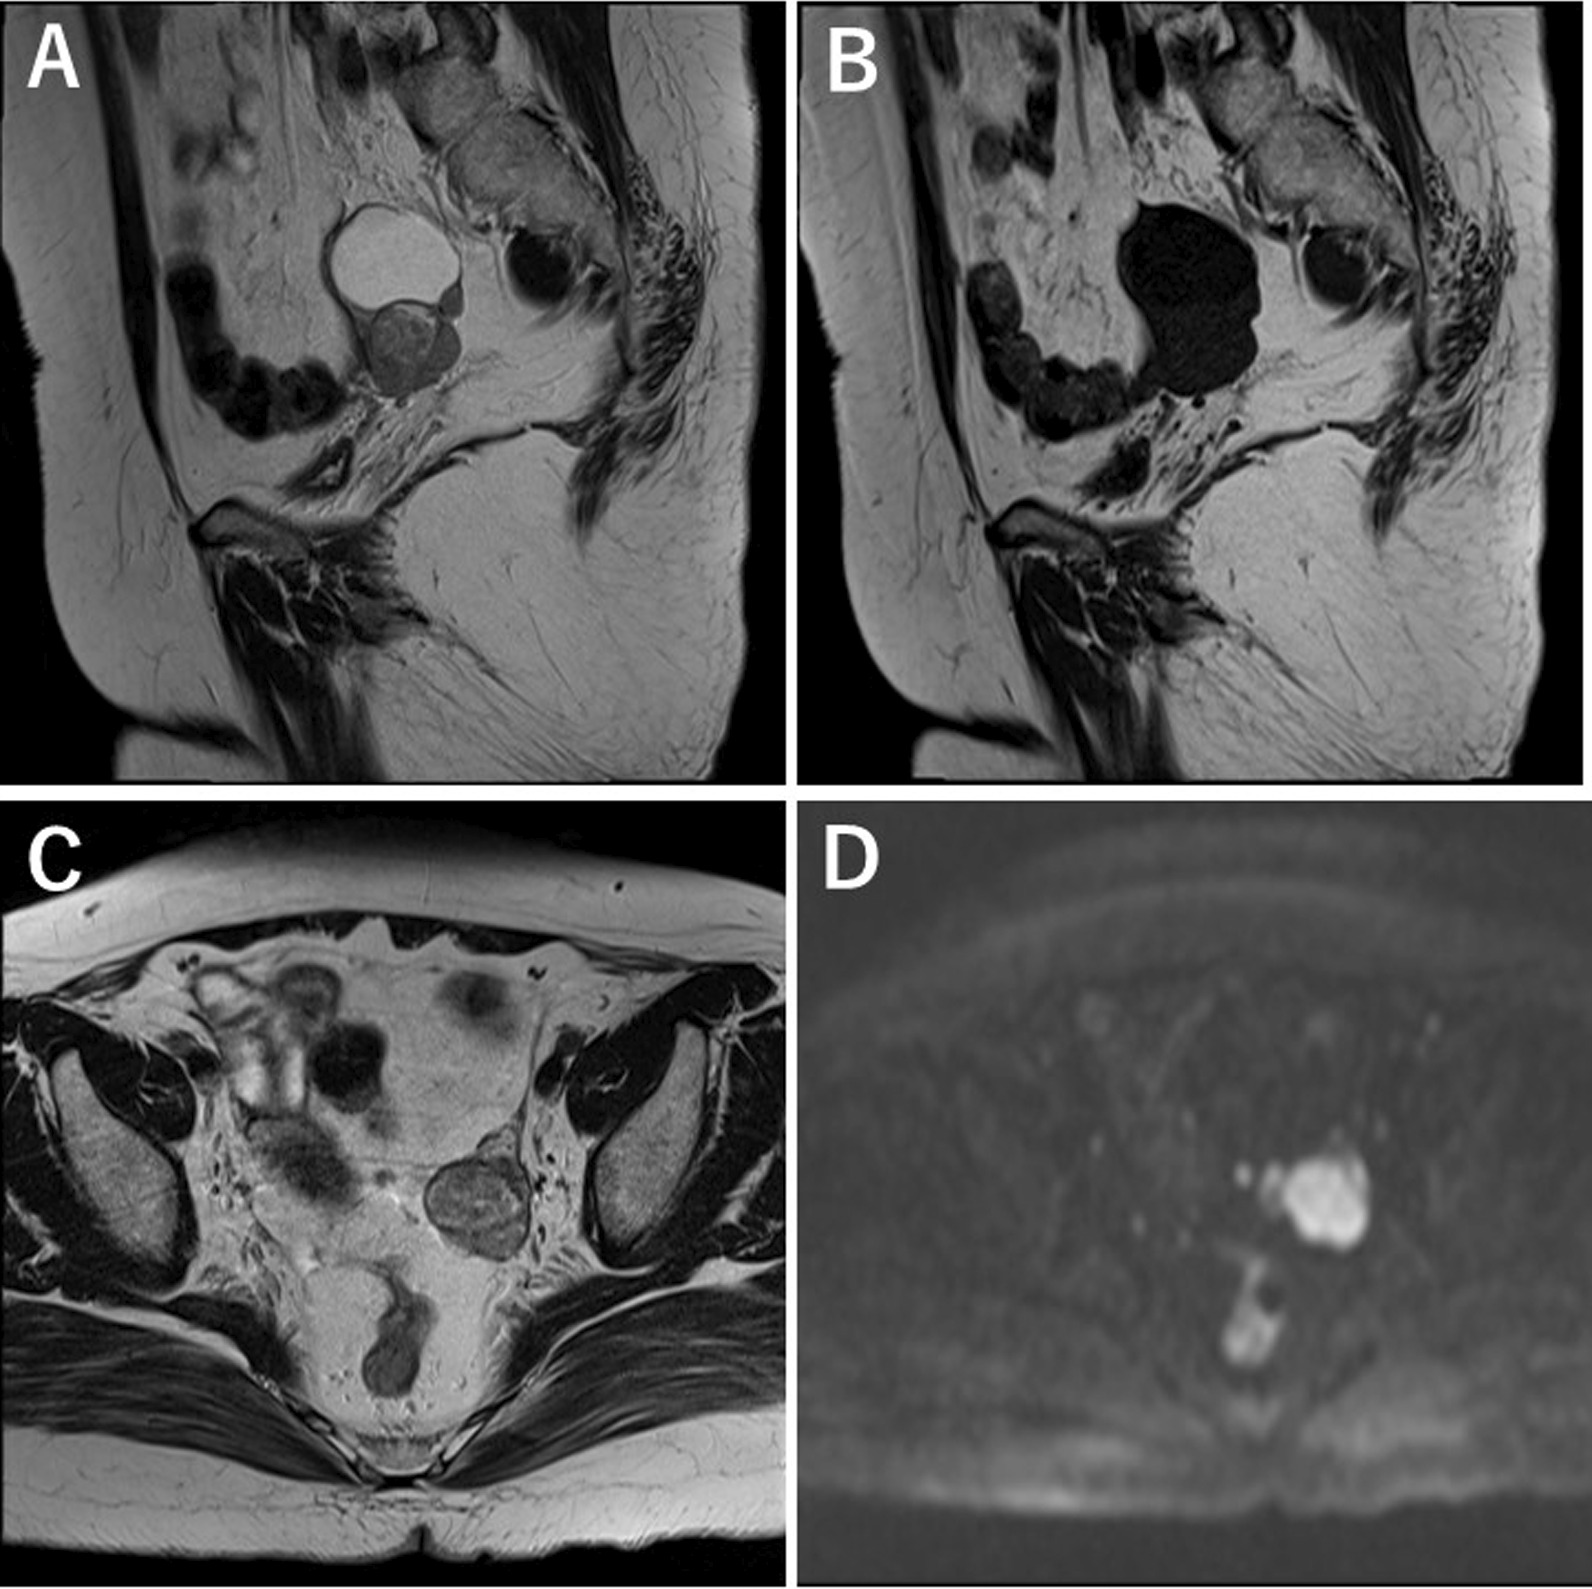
MRI of a mass in the pelvis. A 57 × 41 × 39 mm cystic mass with solid component originating in the left adnexa. A and C T2-weighted MRI; B T1-weighted MRI; D diffusion-weighted images. A and B MRI in sagittal planes. C and D MRI in axial planes. *MRI* magnetic resonance imaging
